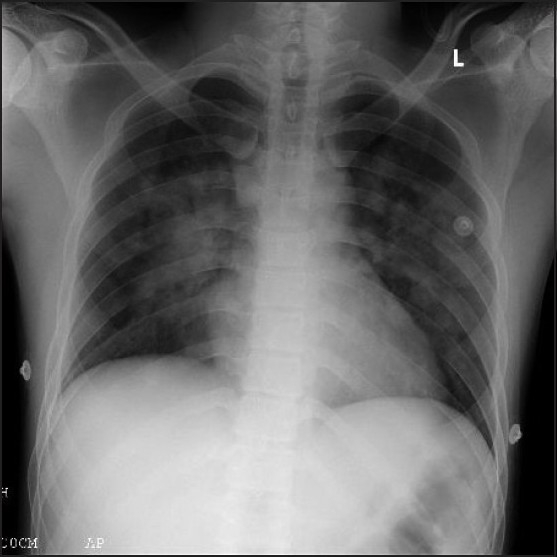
Third post- operative day chest X-ray, showing resolving mid-zone perihilar infiltrates.
